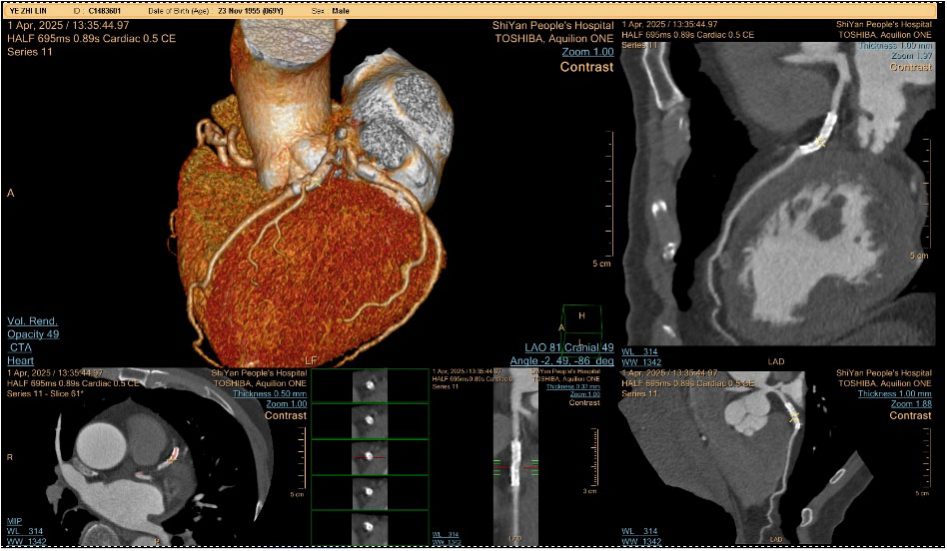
Coronary CTA examination.
